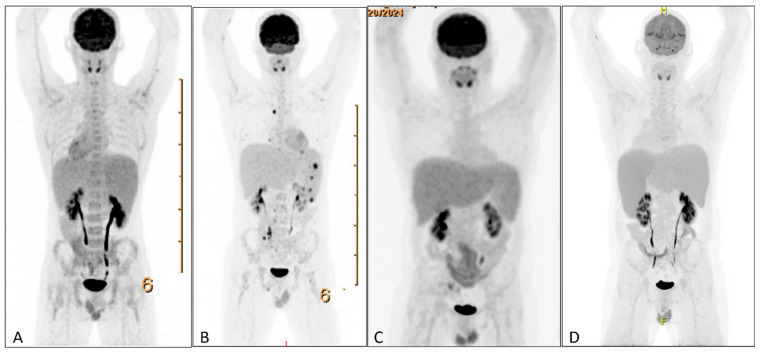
PET scans during clinical course. (A) PET-CT showing splenomegaly without increased FDG-uptake. (B) PET-CT showing additional mesenterial lymphadenopathy, FDG-avid splenic nodules, and bone lesions. (C) PET-CT showing normalization of FDG-avid lymphadenopathy and splenomegaly without increased FDG uptake. (D) PET-CT showing increased splenomegaly with increased FDG uptake. PET = positron emission tomography. FDG = ^18^fluorodeoxyglucose.
